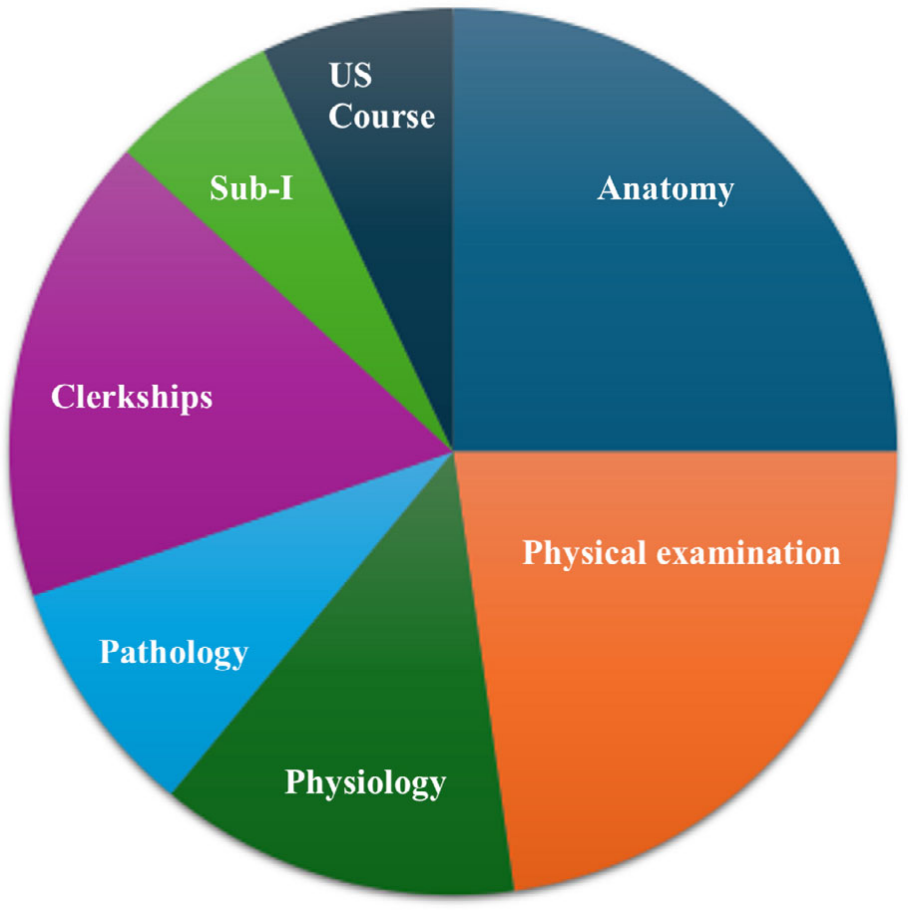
Allopathic medical school courses in 2024 that required ultrasound training.
